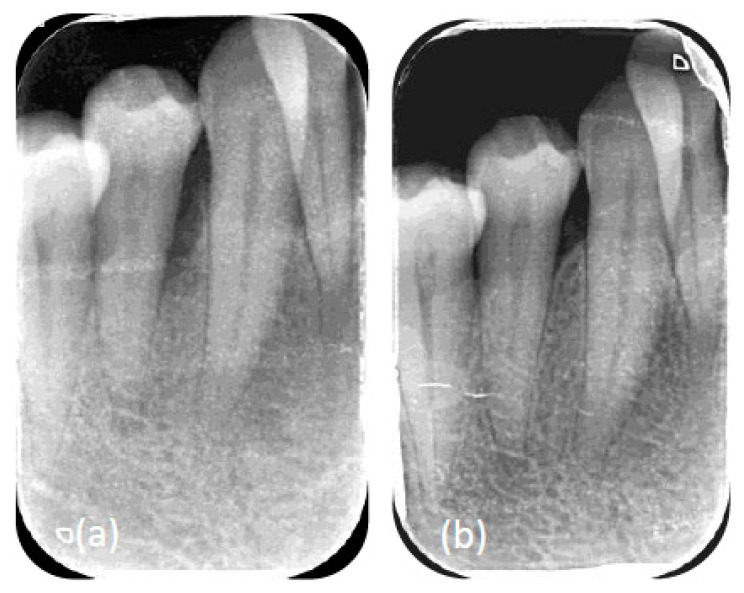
(a) Radiographic view of the angular defect 44 M before regenerative intervention. (b) Radiographic view of the angular defect mesial of the 44 6 months after the intervention, attesting to a good healing capacity after periodontal surgery.
